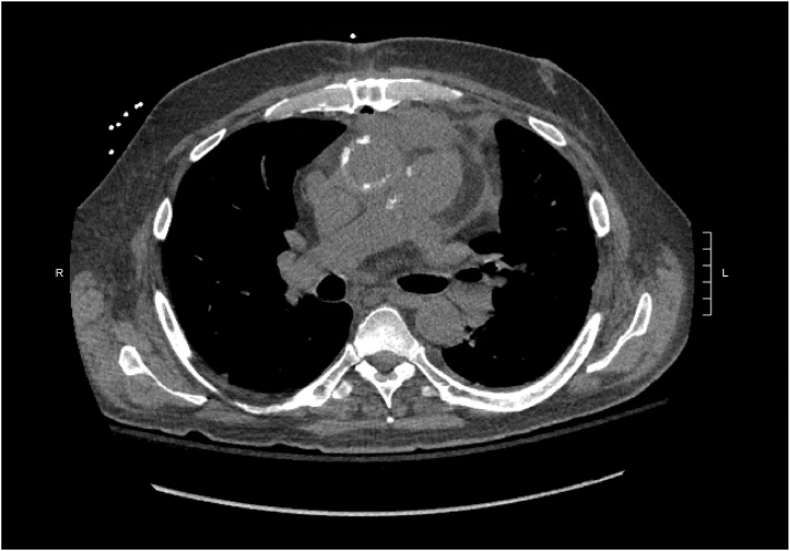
CT scan of the chest demonstrating a six-centimeter retrosternal fluid and gas collection in the operative fie.
